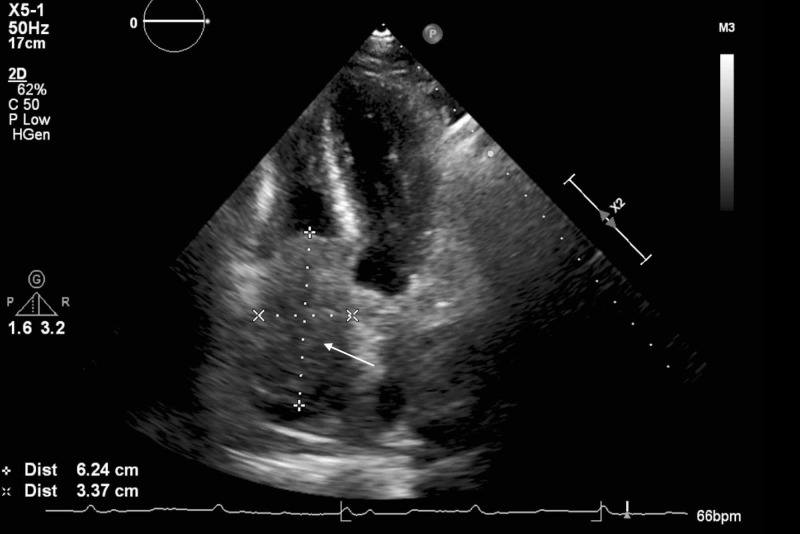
Echocardiogram Echocardiogram revealed the 6 cm x 3 cm mass or thrombus occupying the right atrium with tricuspid involvement. There is no visible extension into the superior vena cava.
